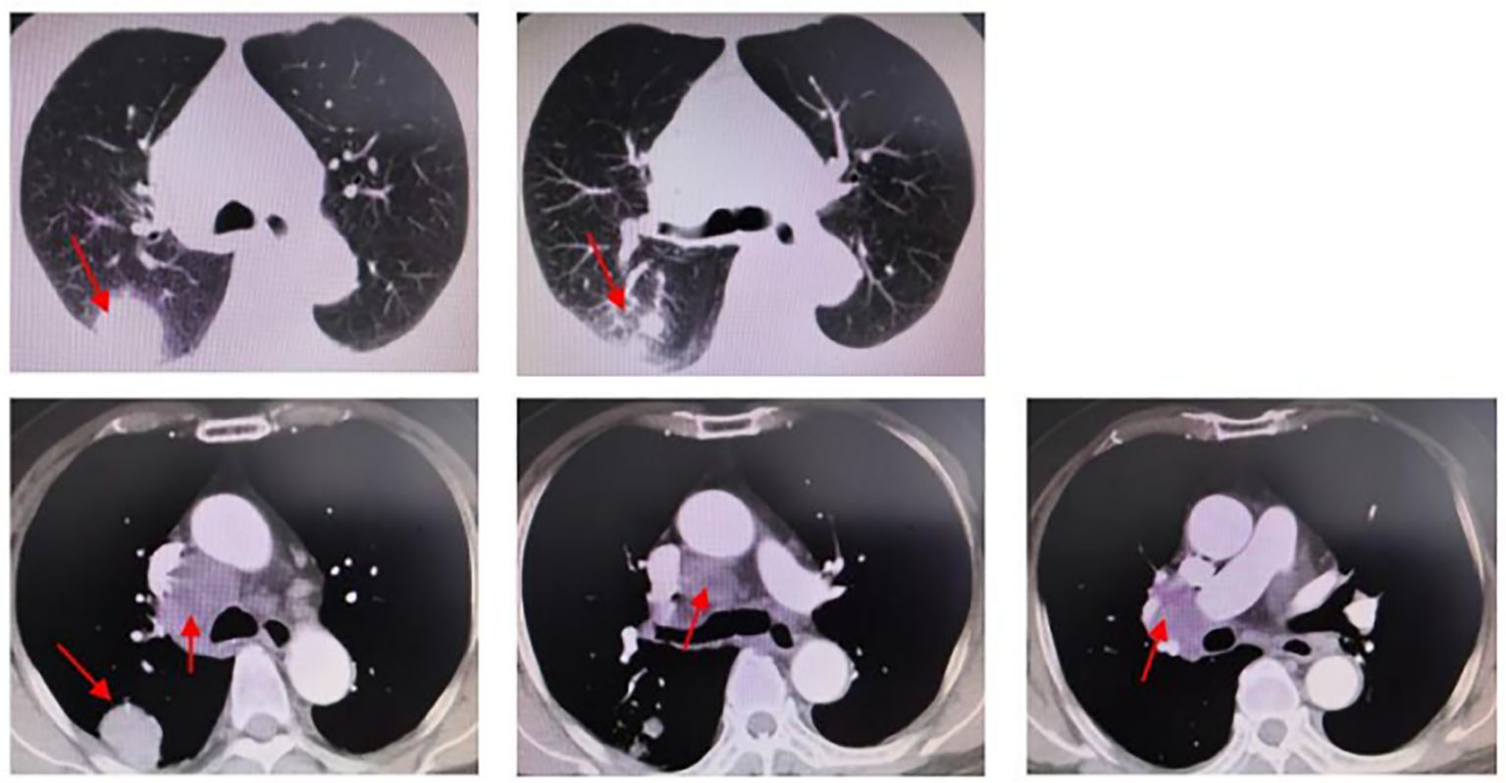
Chest CT (2020.12.18): peripheral lung cancer in the upper lobe of the right lung, approximately 3.39 cm in diameter. Right hilar and mediastinal lymph node metastasis; the larger one is approximately 4.47cm in diameter.
